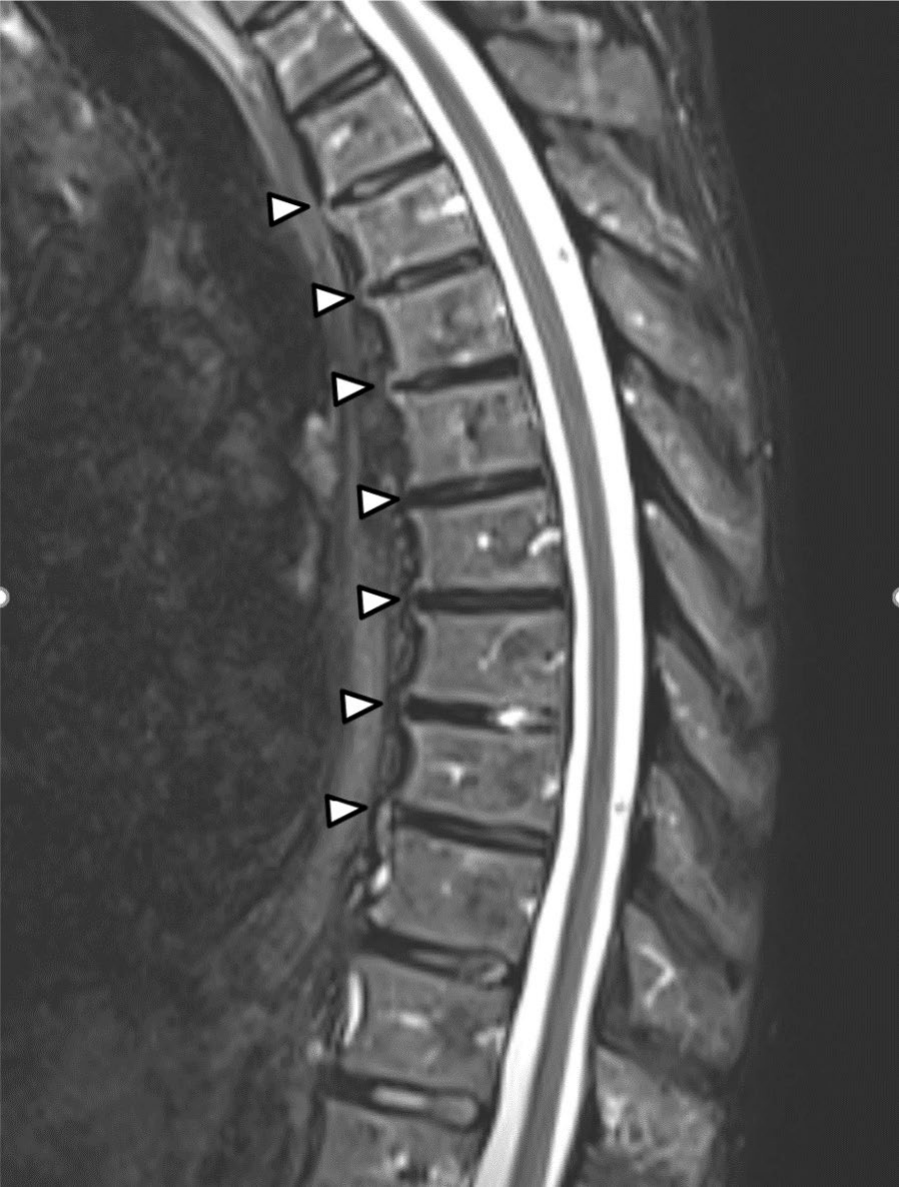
MRI (TIRM sequence) of the thoracic spine. Image in the sagittal plane in a patient (male, 61 years old) diagnosed with axial SpA. Multiple thoracic anterior syndesmophytes are depicted (white arrowheads)
